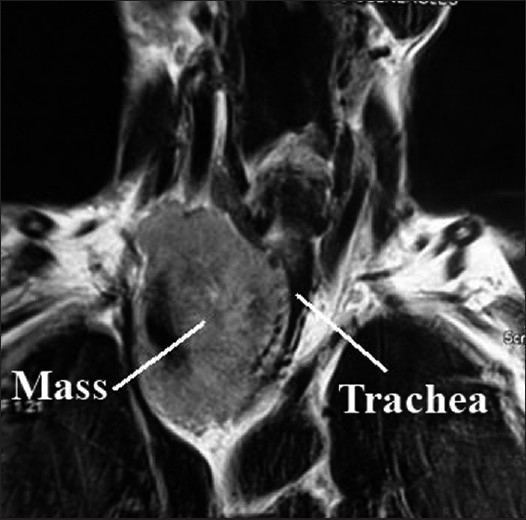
Cross-sectional view of MRI showing the trachea and tortuosity of the mass
